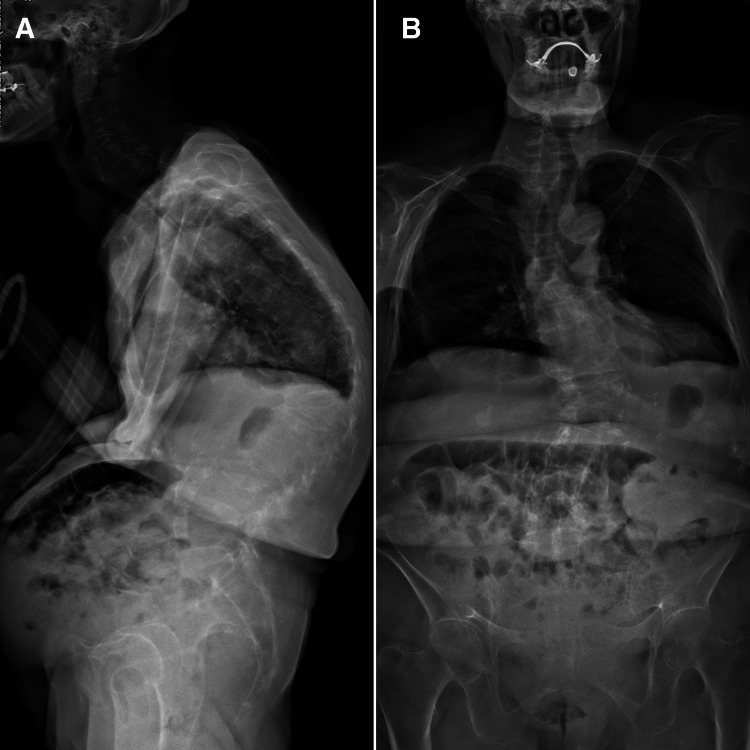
Full-spine radiograph. (A) Generalized decreased bone density is indicative of osteopenia/osteoporosis and renders assessment suboptimal at the frontal view. Degenerative changes with marginal osteophytes in the thoracic and lumbar spine. Compression fracture from T11-L1 with marked loss of vertebral height. Mild loss of vertebral height is seen in the L3 vertebral body. Collapse of T9 was also seen. These are likely osteoporotic in nature. (B) Moderate thoracolumbar scoliosis with convexity to the left. Some of the vertebral bodies cannot be well assessed on the lateral view.
